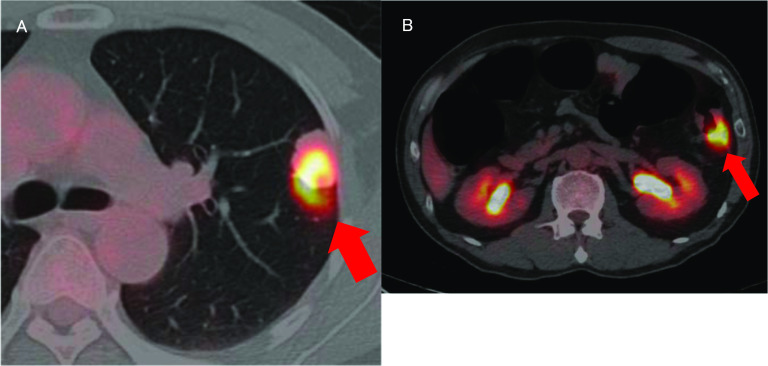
Positron emission tomography (PET)–CT findings. A PET–CT revealed a standardized uptake value (SUV) max of 9.2 fluorodeoxyglucose (FDG) accumulation in S3a of the left upper lung lobe (red arrow). B PET–CT revealed a SUV max of 9.8 FDG accumulation in the descending colon (red arrow)
